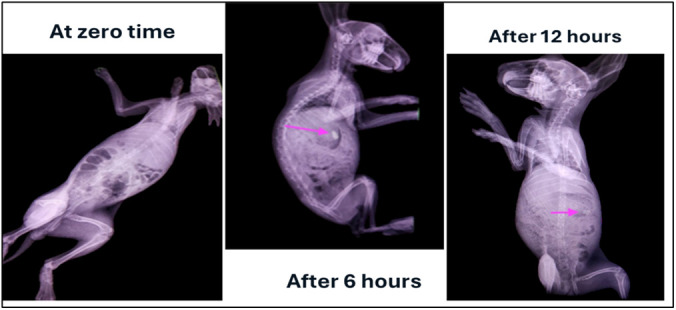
Radiographic images showing the gastric retention of the optimized formulation (F14) at different time intervals after administration, demonstrating its expansion and persistence in the stomach.
